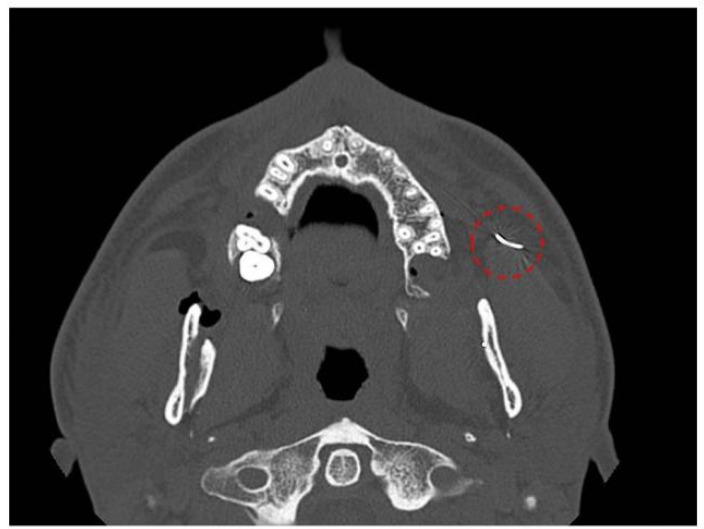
Axial computed tomography (CT) image demonstrating the presence of a suture needle within the left masticatory space. The foreign body is indicated by a dotted circle.
